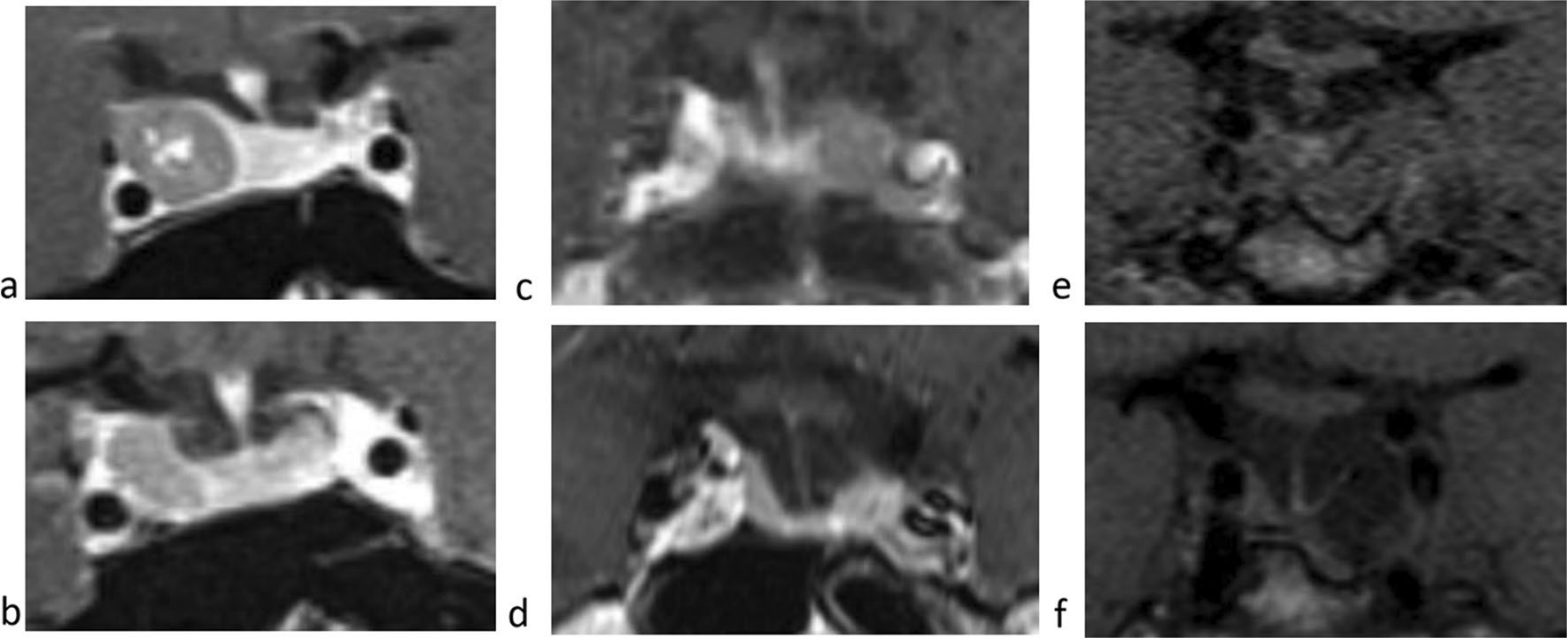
Pituitary MRI before (a), and after (b) 5-years therapy with PEG and SRLs combined therapy (patient not previously subjected to neurosurgery); before (c), and after (d) 10-years PEG monotherapy; before (e), and after (f) 10-years therapy with PEG and SRLs combined therapy
